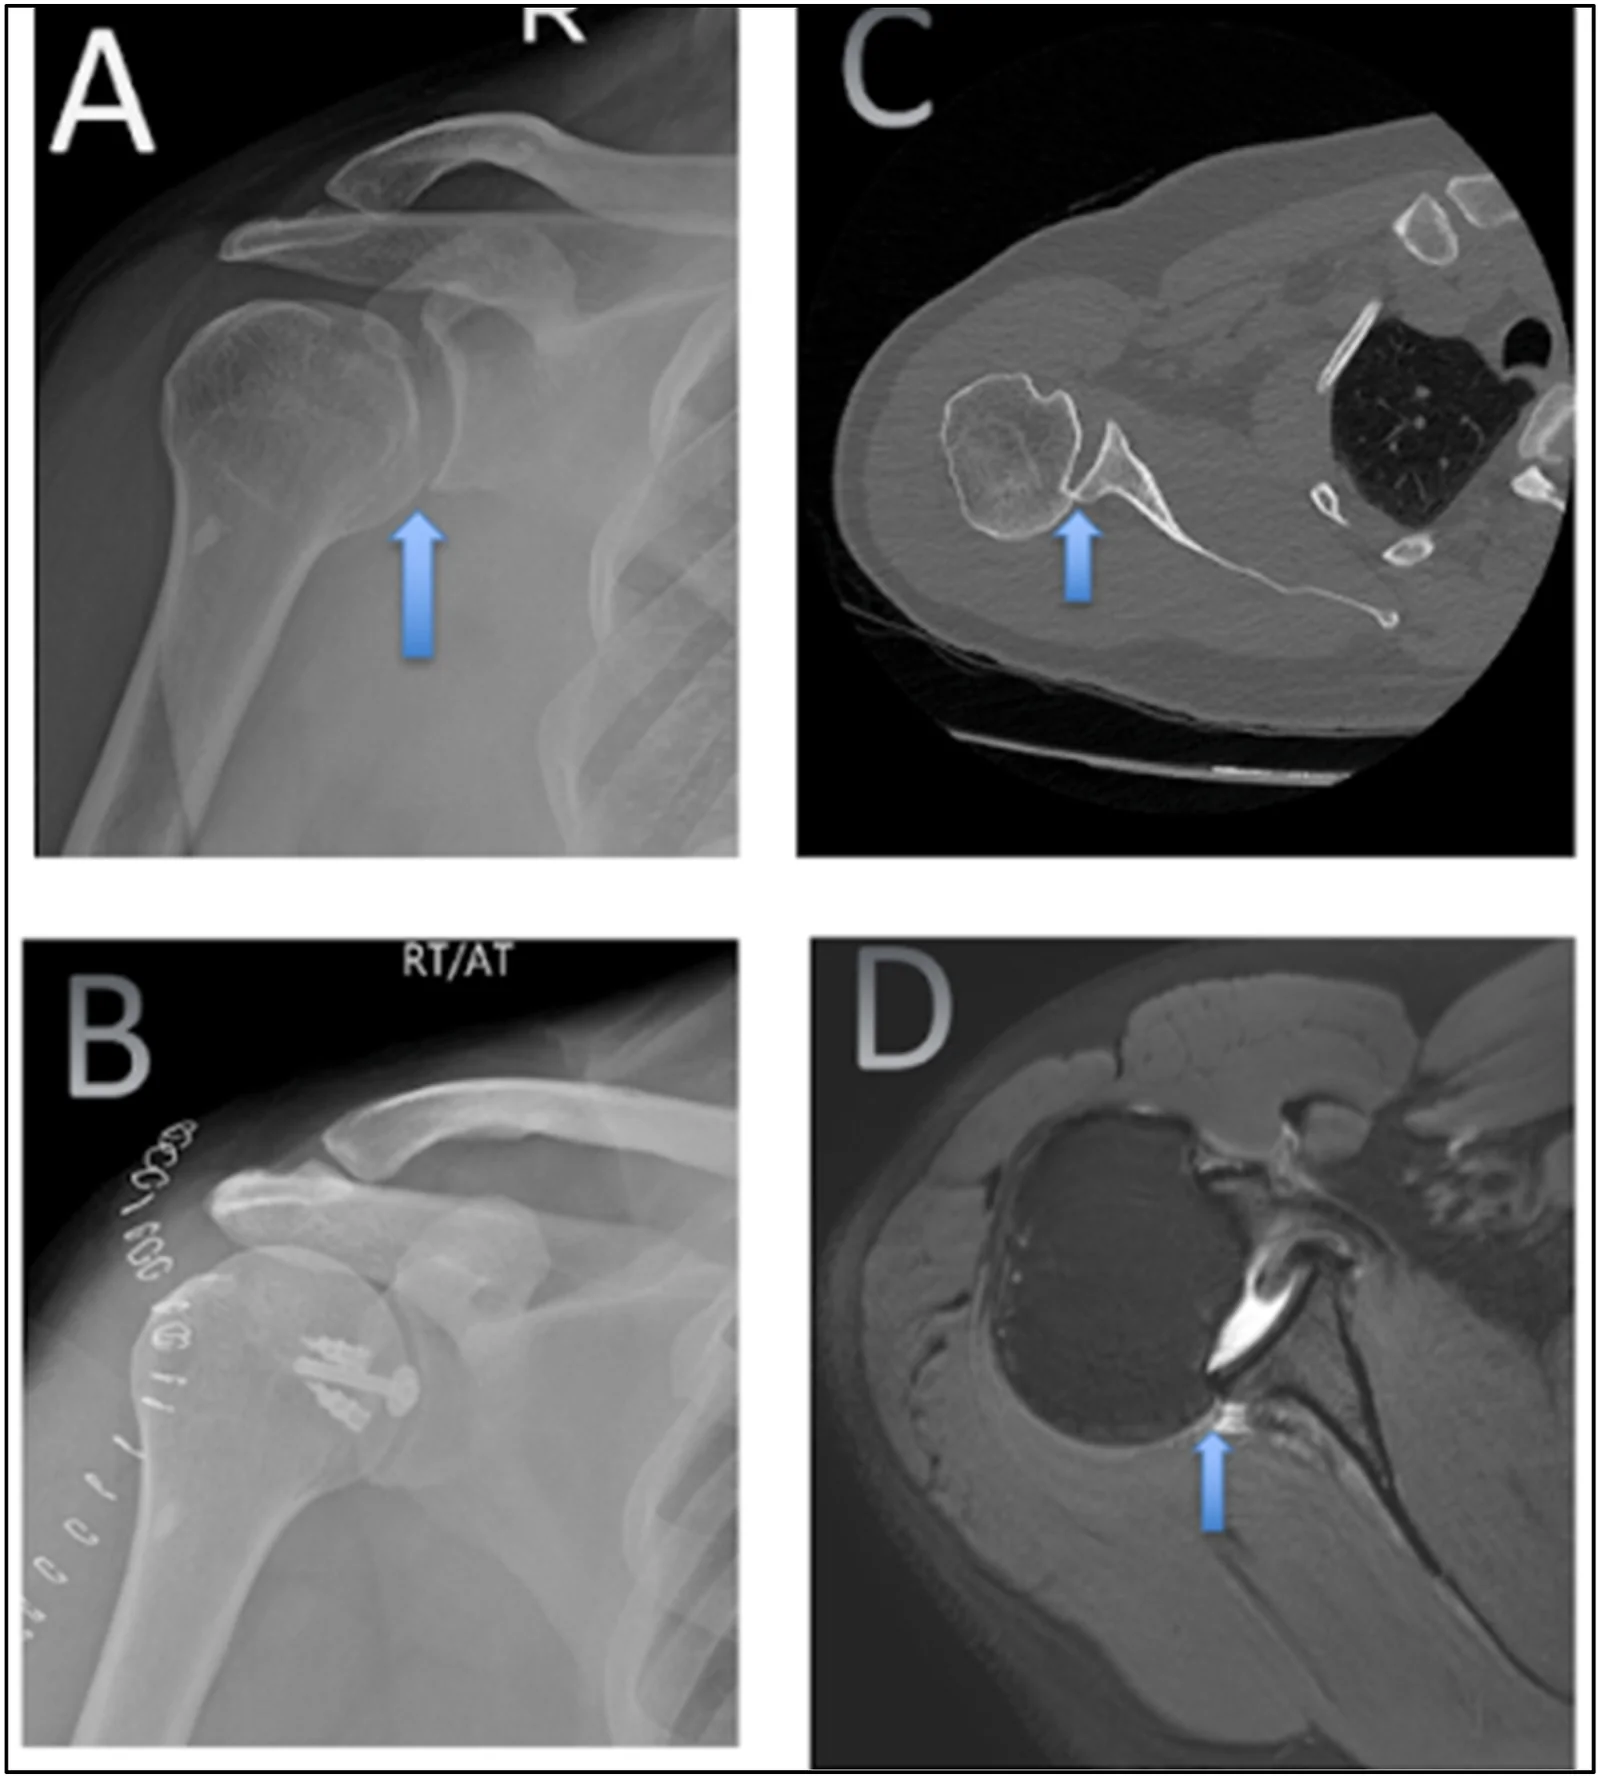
A) Anterior-posterior (AP) view showing dislocated shoulder with incongruous joint line (and light bulb sign); B) Postoperative radiograph showing internal fixation of the lesser tuberosity (Modified McLaughlin's procedure); C) CT image showing posterior dislocation with a humeral head defect; D) MRI (PDFS – proton density fat suppressed axial) image showing posterior dislocation with a humeral head defect (reverse Hill-Sachs lesion).
